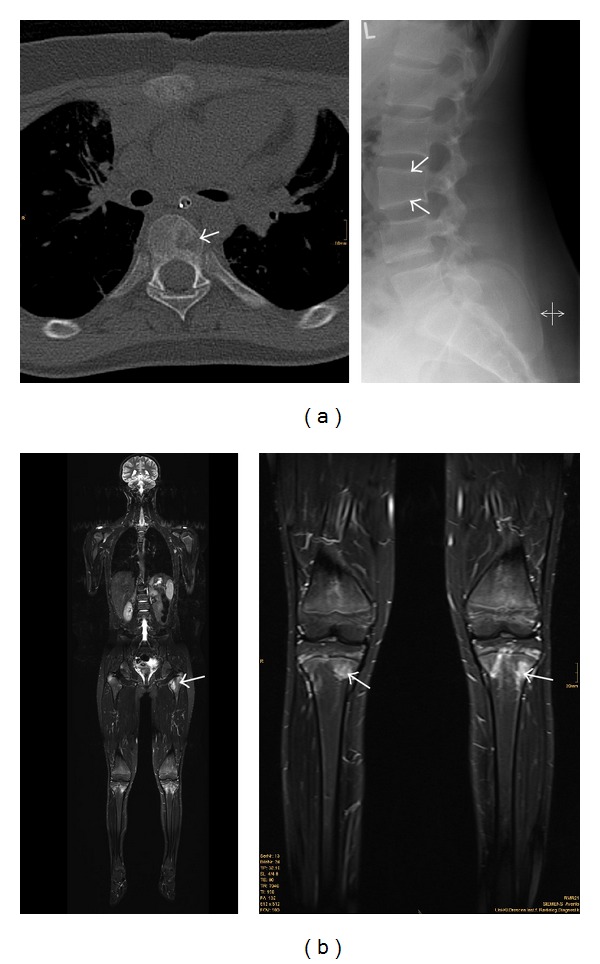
(a) left panel: computerized tomography (CT) of the spine, showing an osteolytic lesions (white arrow) in chronic recurrent multifocal osteomyelitis in a thoracic vertebra. Right panel: radiograph of the spine, showing flattening of the third lumbar vertebra (white arrows) in chronic recurrent multifocal osteomyelitis. (b) magnetic resonance imaging (MRI) in a patient with chronic recurrent multifocal osteomyelitis; left panel: whole-body MRI, showing multiple foci of osteomyelitis, some of which are distributed symmetrically; right panel: magnified image (from the left panel) of the knees, showing inflammatory epiphyseal lesions in both tibiae.
